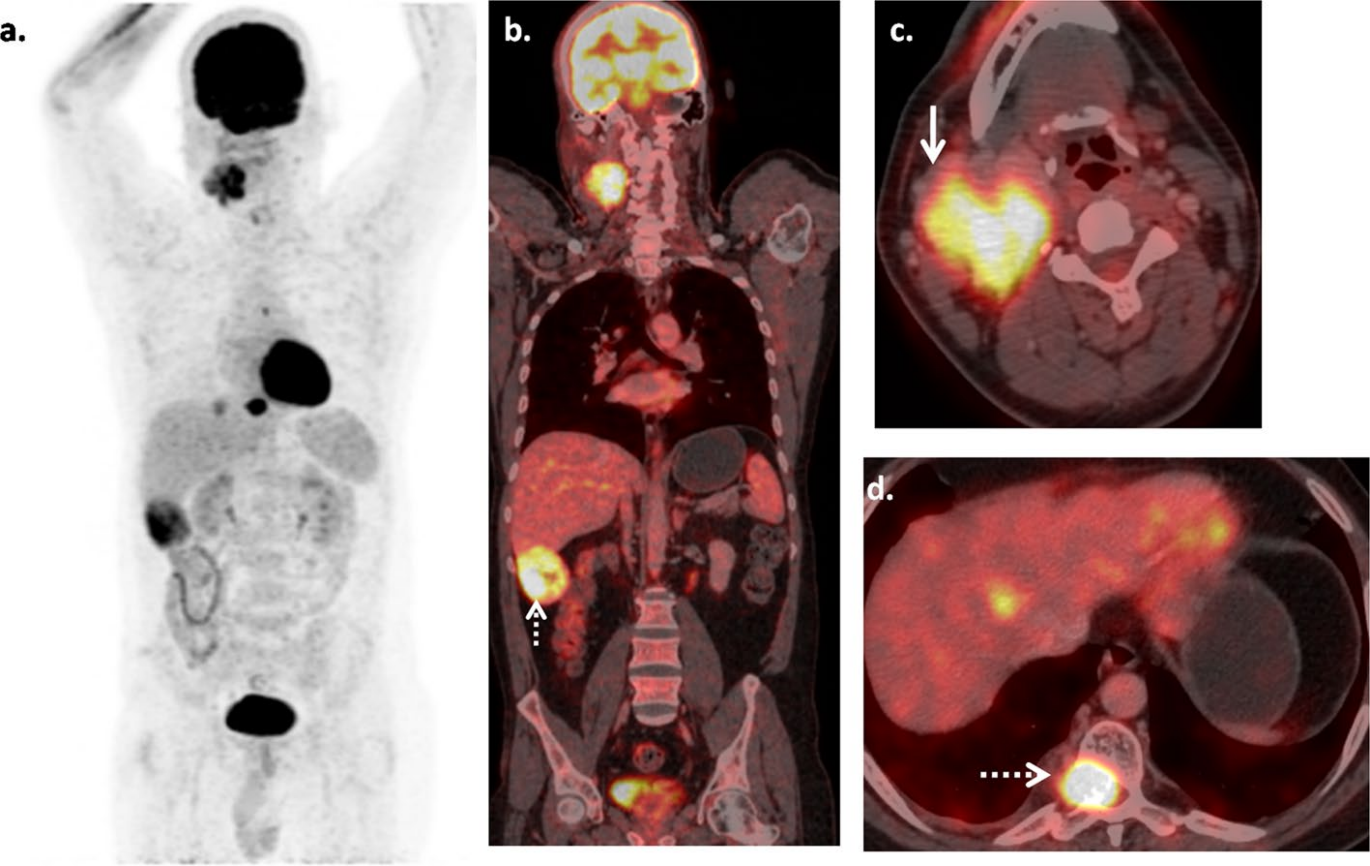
The Maximum intensity projection (a) and fused coronal PET/CT (b) and axial (c) images show abnormal FDG uptake in right carotid space causing splaying of the carotid arteries with their encasement (white arrows). Note is made of the metabolically active hepatic lesion in segment V–VI (dotted arrows) in fused coronal image (b) and lytic lesion in D9 vertebrae (dotted arrows) in fused axial image (d)
